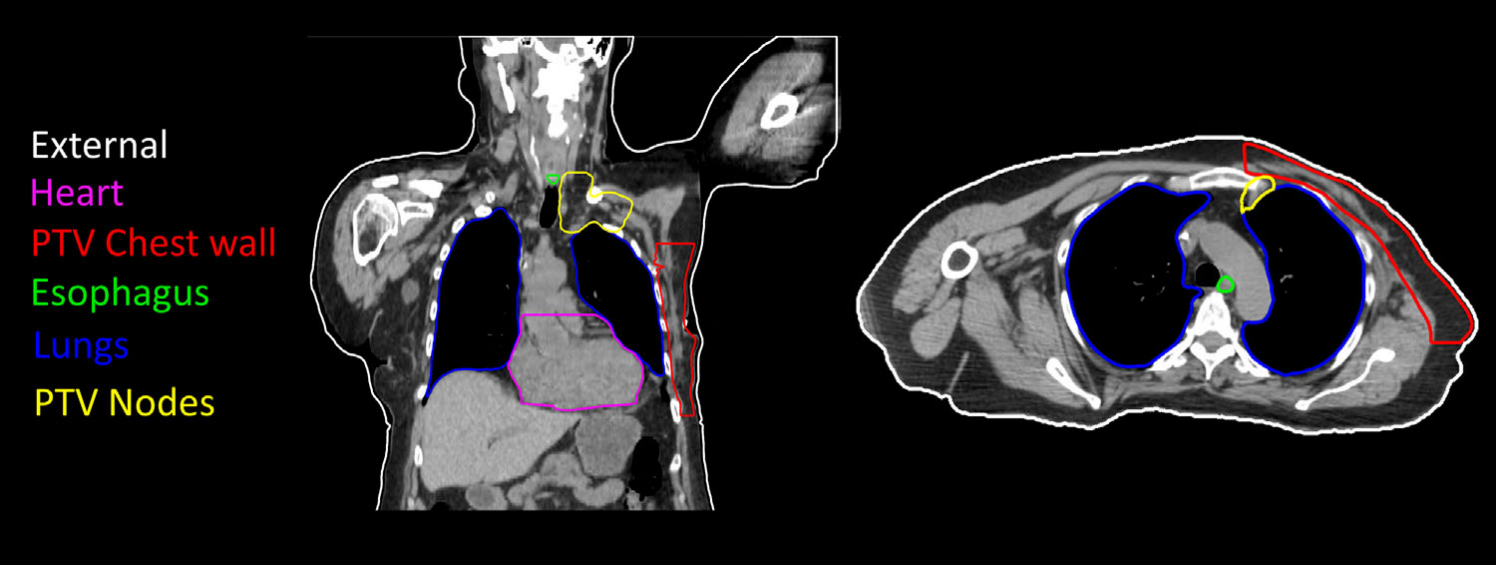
CT scan slices of a patient receiving PBS irradiation of the chest wall with the following contoured targets and OARs: heart (purple), PTV chest wall (red), esophagus (green), lungs (blue), and PTV nodes which includes the axilla, supraclavicular lymph nodes and internal mammary chain (yellow).
